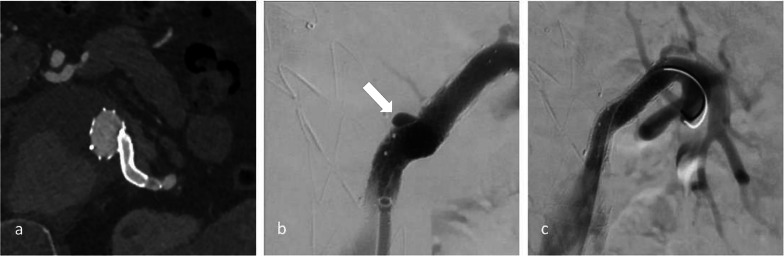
In the same procedure as in Fig. 1, the left renal BSG was unsuspicious in CTA (a), but an incidental membrane disruption was detected at angiography (b) and successfully treated (c)
